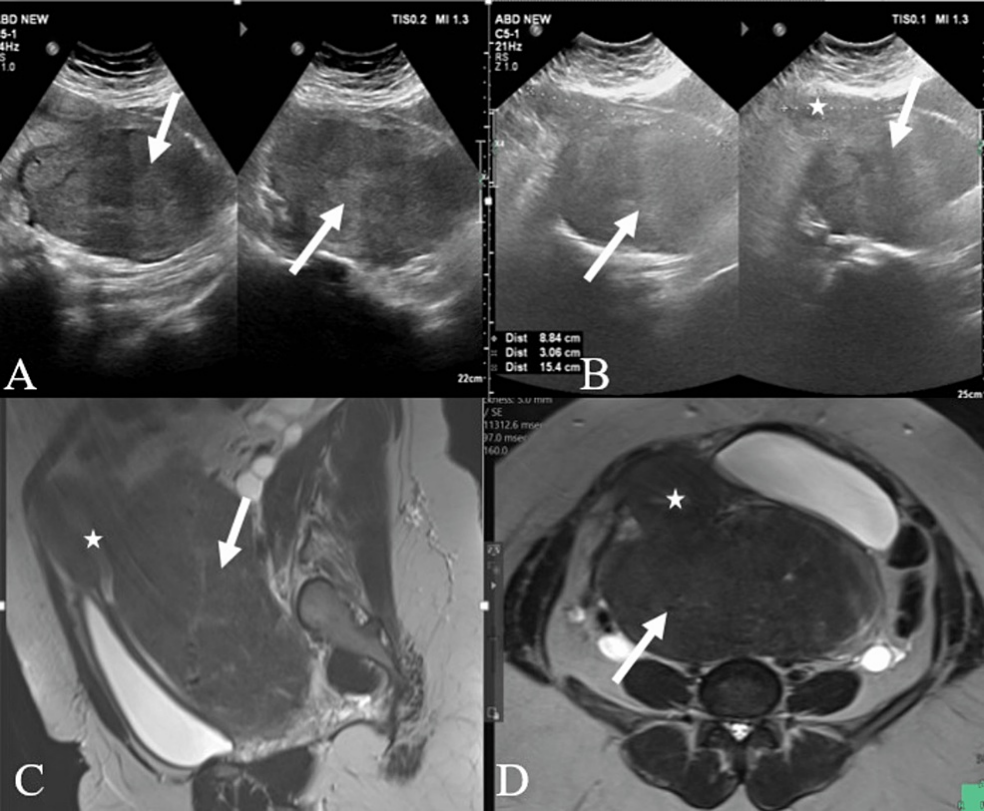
A case of large cervical fibroid A: USG pelvis transverse section showing a large heterogeneously iso-hypoechoic lesion in the pelvic cavity (white arrow). B: USG pelvis longitudinal section showing a large heterogeneously iso-hypoechoic lesion (white arrow) in the pelvic cavity pushing the uterus (star) anteriorly. The cervix is not visualized separately. C: Sagittal T2-weighted pelvis MR image showing heterogeneously hypointense lesion (white arrow) in pelvis arising from the cervix, consistent with cervical fibroid. D: Axial T2-weighted pelvis MR image showing heterogeneously hypointense lesion (white arrow) in pelvis arising from the cervix, pushing the uterus (star) anteriorly, consistent with cervical fibroid. USG -  ultrasonography
